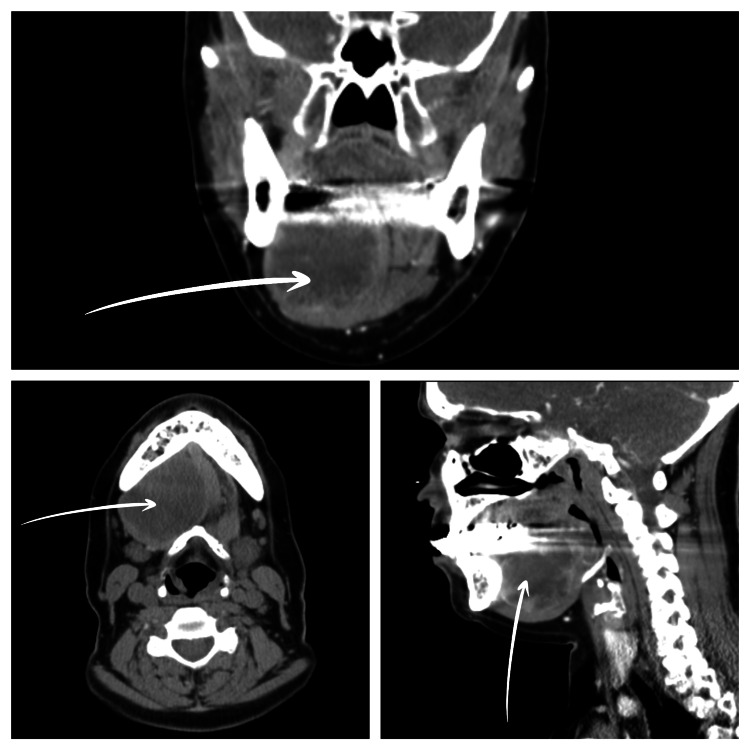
Contrast-enhanced CT images show a right-side expansive lesion centered on the floor of the mouth. Upper: coronal. Lower left: axial. Lower right: sagittal
